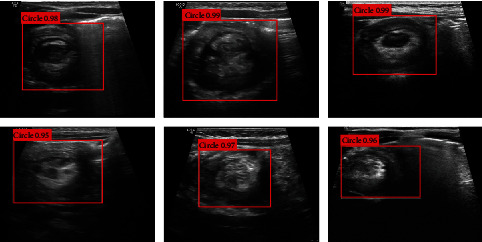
Ultrasound images of pediatric intussusception in the validation set.
